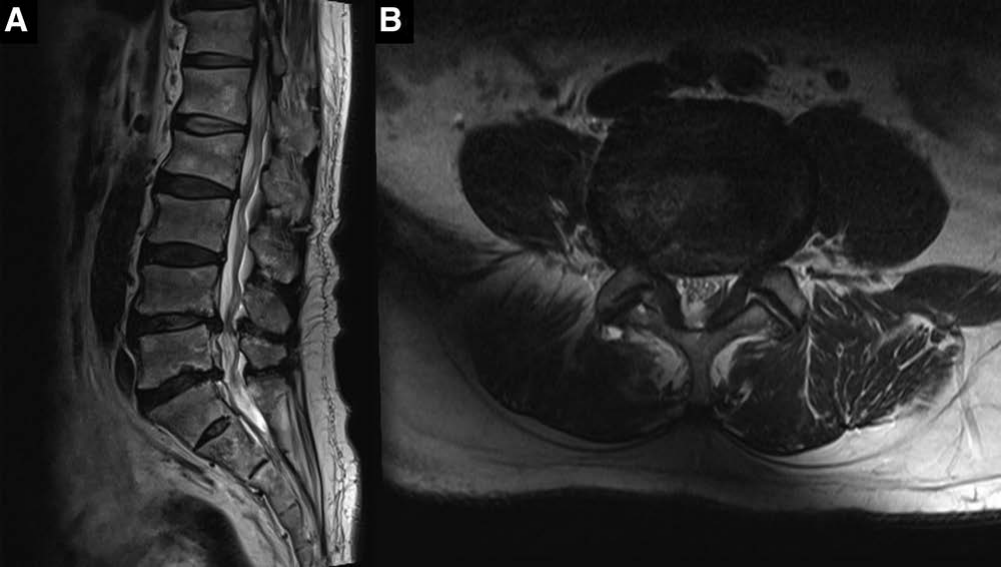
(A) Sagittal and (B) axial T2-weighted magnetic resonance images taken prior to the decompressive laminectomy showing spinal stenosis at the L4 to 5 level.
